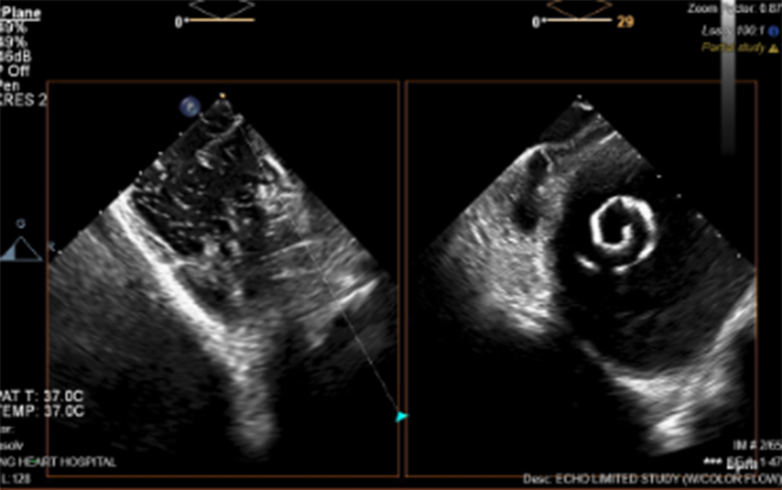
Intracardiac Echocardiography Visualization of Safari Wire Within the LV Cavity Visualization of equipment during this case was imperative to minimize instrumentation of the left ventricular (LV) thrombus.
